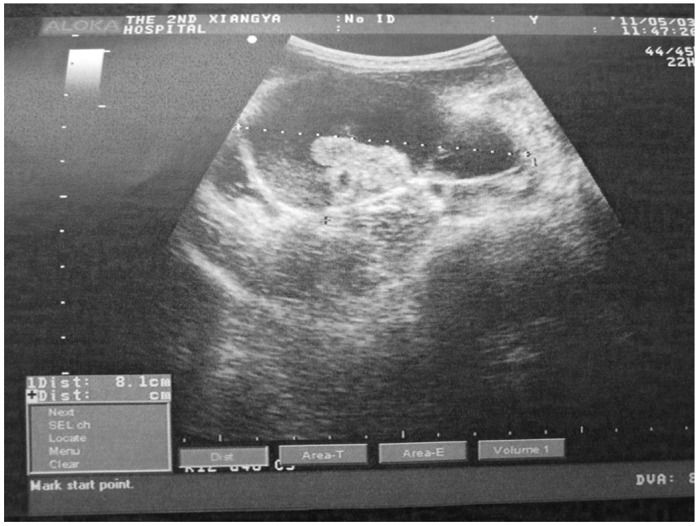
Sonographic examination of the pelvis revealed that the mass contained foci of calcification of ∼3×2 cm.
